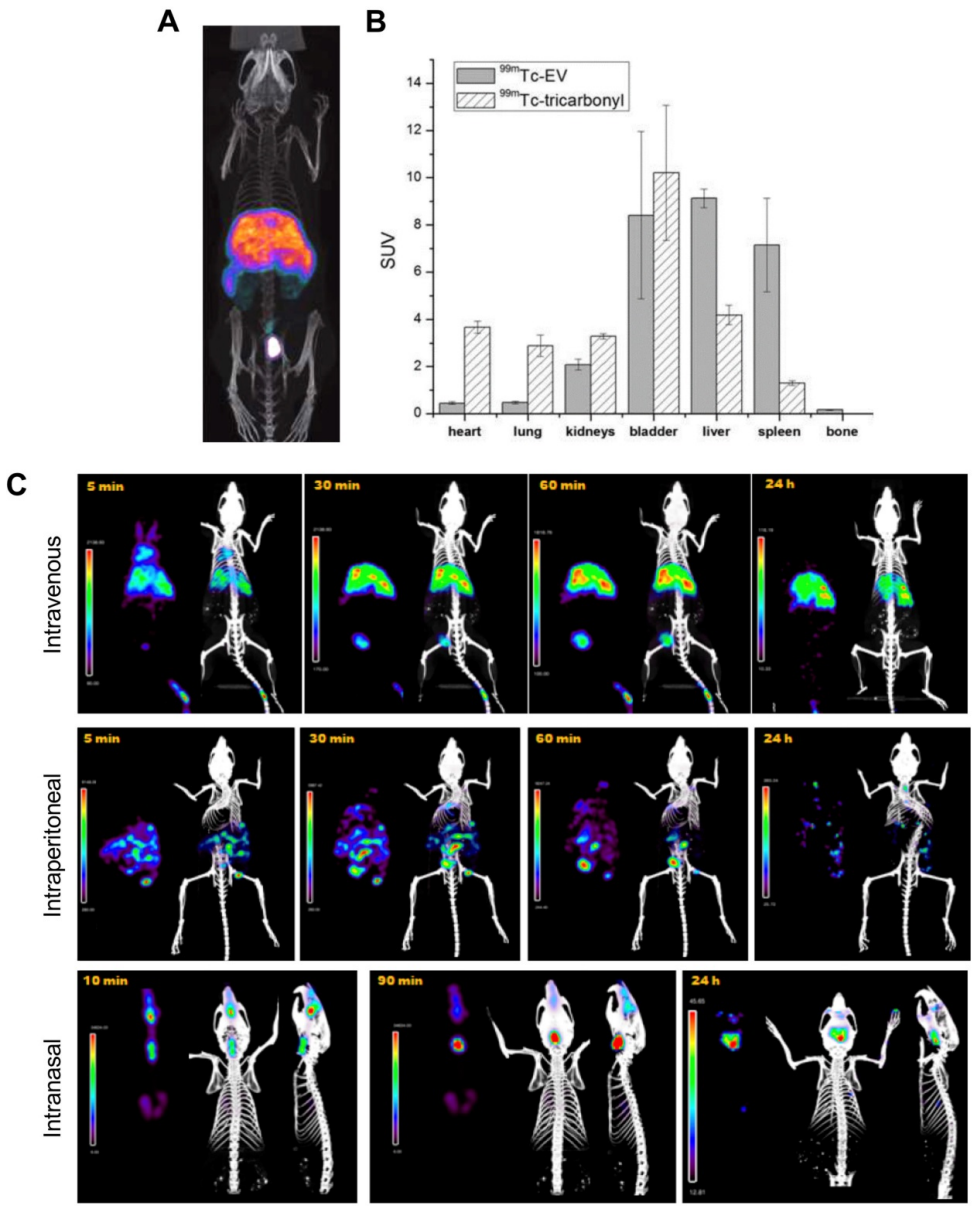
A) SPECT-CT images of [^99m^Tc]Tc-tricarbonyl labelled RBC derived sEVs. B)
